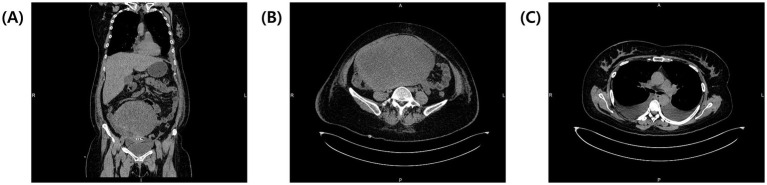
Post-NACT CT of the thorax and abdomen. (A) Coronal view showing the enlarged tumor compressing surrounding structures. (B) Transverse view of the abdomen with enlarged tumor now measuring 20.5 × 13.5 × 17.3 cm. (C) Transverse view of the thorax showing bilateral pleural effusions at the level of tracheal bifurcation.
